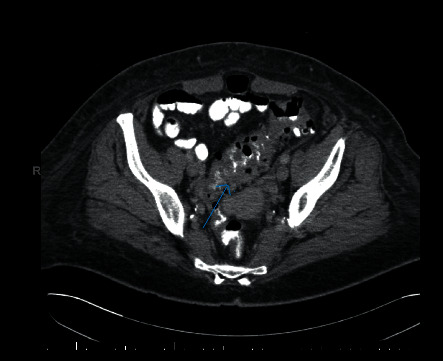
Axial computed tomography of the abdomen and pelvis demonstrating sigmoid colonic thickening and mild sigmoid colitis with no evidence of perforation on day 2 of hospitalization (blue arrow).
